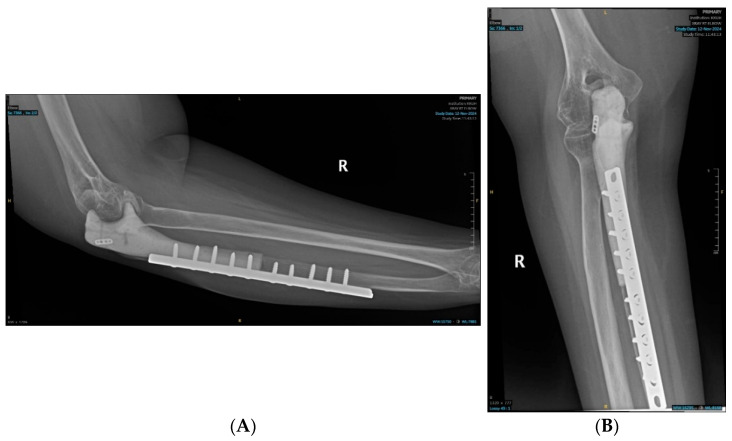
Postoperative radiographs of the ulnar reconstruction: (A) Ulnar implant—lateral view; (B) Ulnar Implant—Anteroposterior view.
